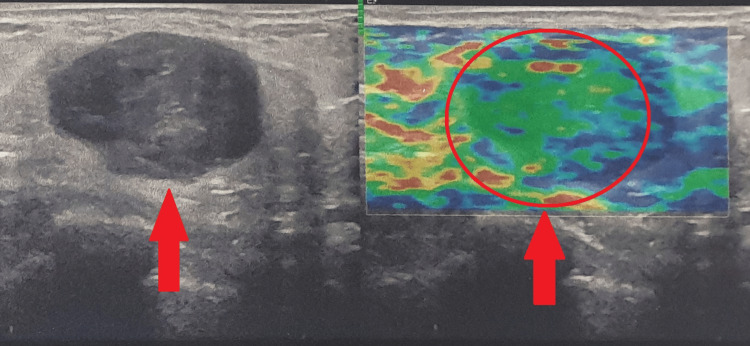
Ultrasound findings. Grayscale ultrasound image demonstrates a large, heterogeneous, predominantly hypoechoic mass with mildly irregular and smooth margins and possibly areas of internal necrosis (left red arrow). Corresponding real-time strain elastography map of the same region with increased stiffness (right red arrow area).
